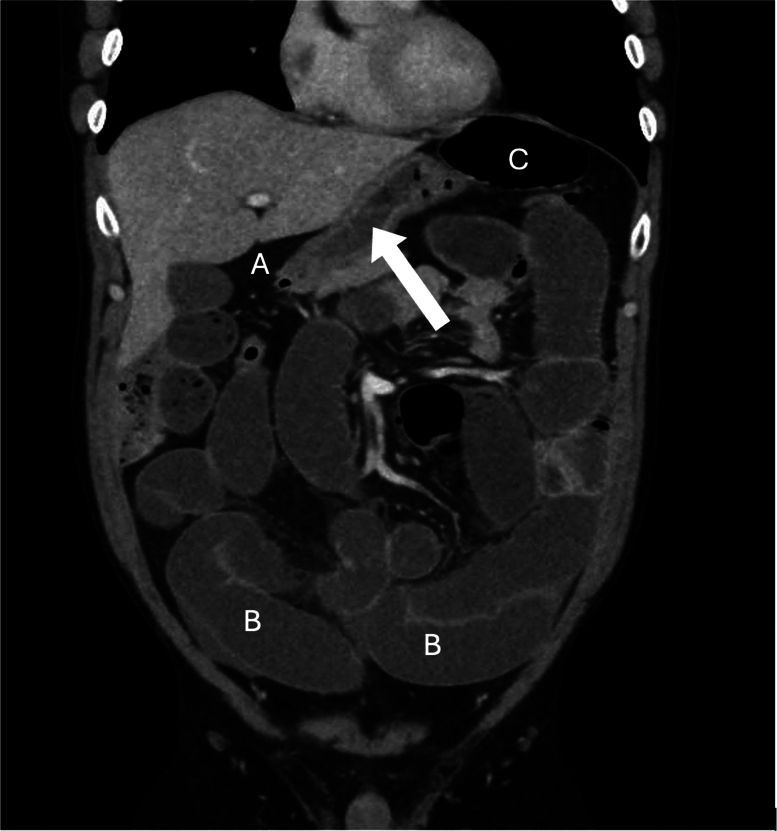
Coronal section of a computed tomography scan showing terminal ileum herniation through the foramen of Winslow (A) with displacement of the stomach (B) and dilated small bowel loops (C). The white arrow denotes terminal ileum herniation through the foramen of Winslow.
